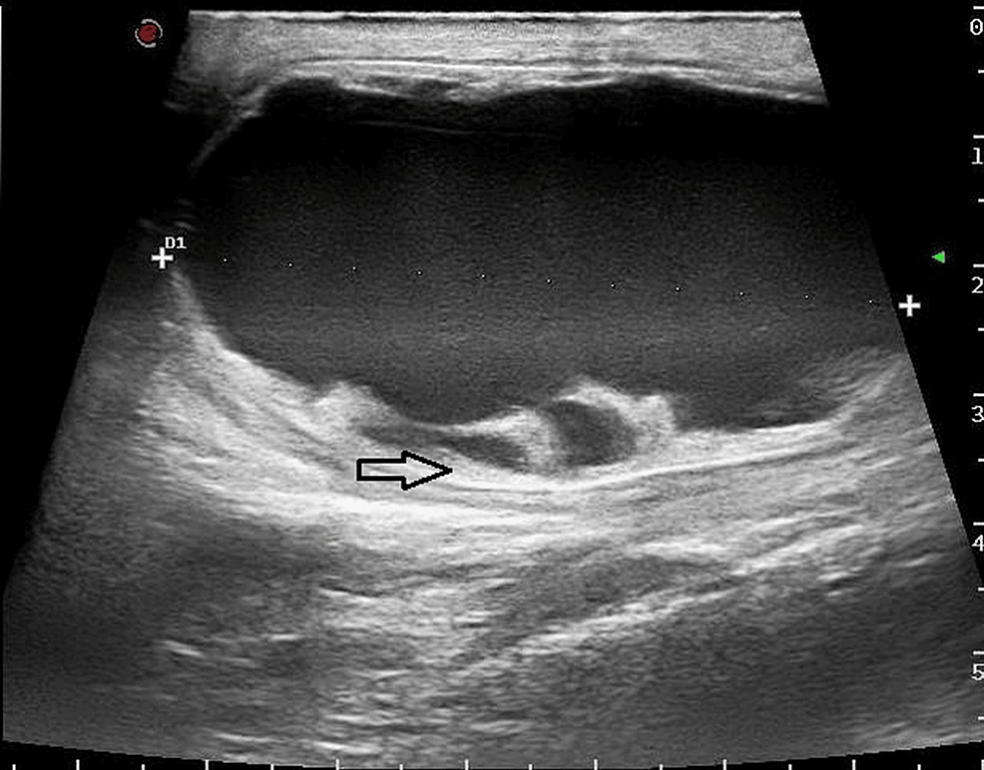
Transverse ultrasound view of the left epididymal cyst with fine internal echoes and thin septae.
